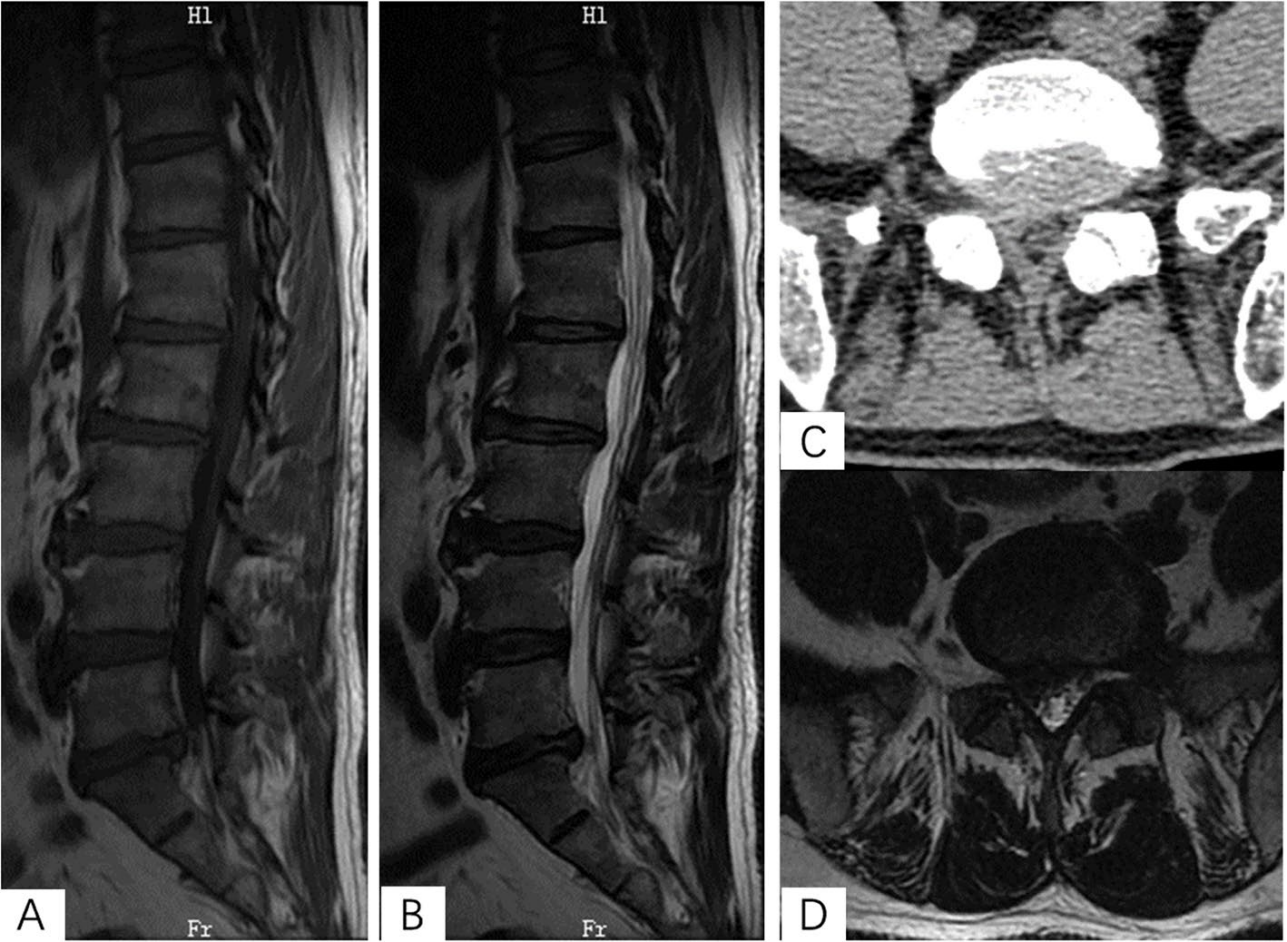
A 44-year-old male with L5/S1 lumbar disc herniation underwent PLED with Robot Assistance. A, B and D) Preoperative MRI images. C) Preoperative CT scan image
